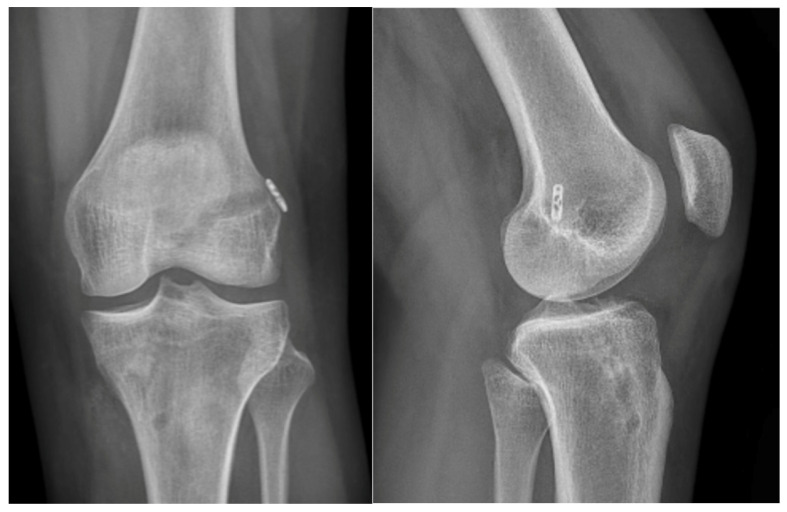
Standard postoperative radiographs after ACL + ALL reconstruction. (Left) Weight-bearing anteroposterior view. (Right) Strict lateral view.
